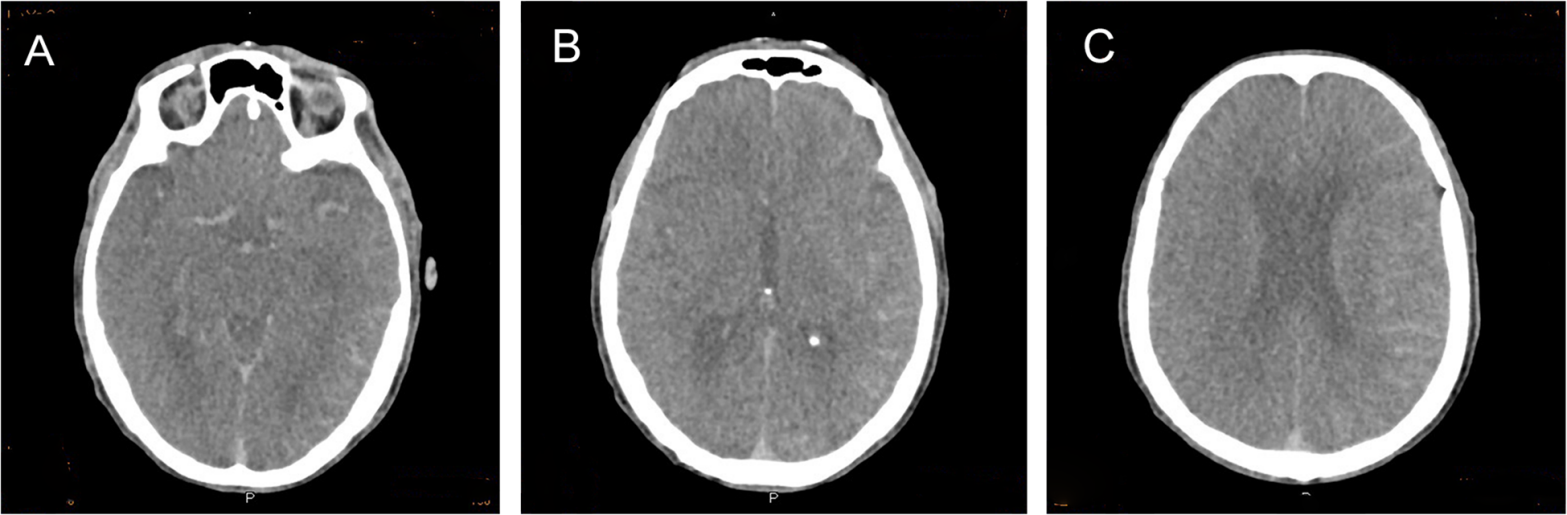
CT scan of the patient’s head at onset; a-c: Swelling of the left cerebral hemisphere and left linear high-density area
